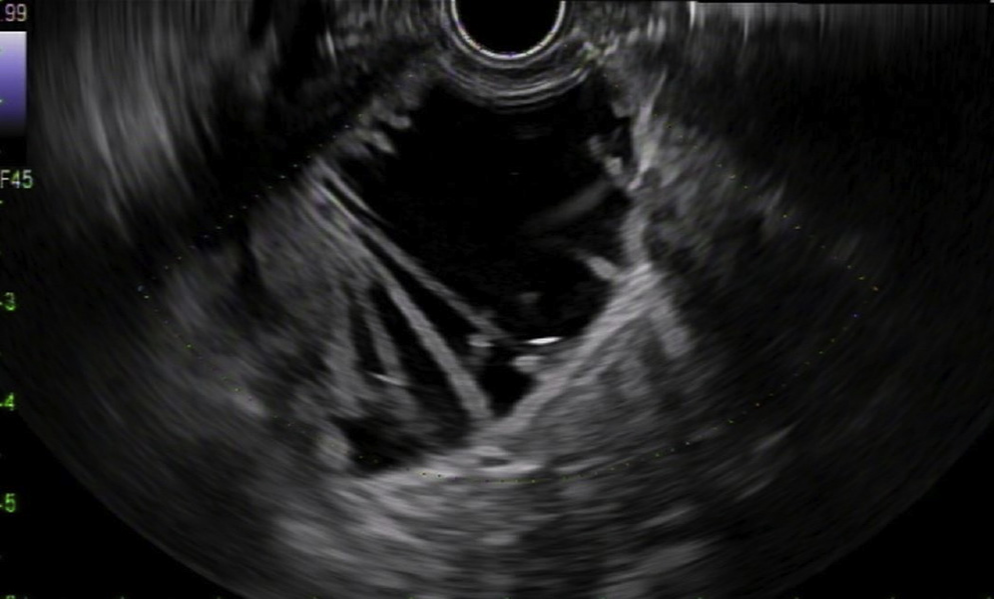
Endosonographic visualization of contrast filled jejunal limb for creation of gastrojejunostomy.
